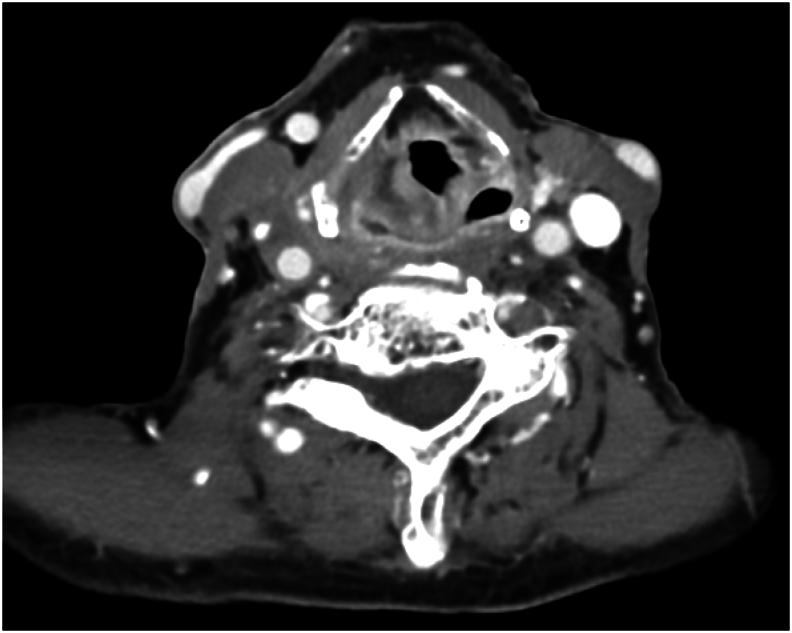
Axial CT showing improvement in primary lesion and neck adenopathy.
